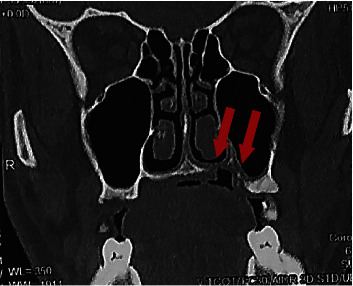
Cone-beam computed tomography (coronal cut) showing the maxillary defect, connecting the oral cavity to the left maxillary sinus and the nasal fossae.
